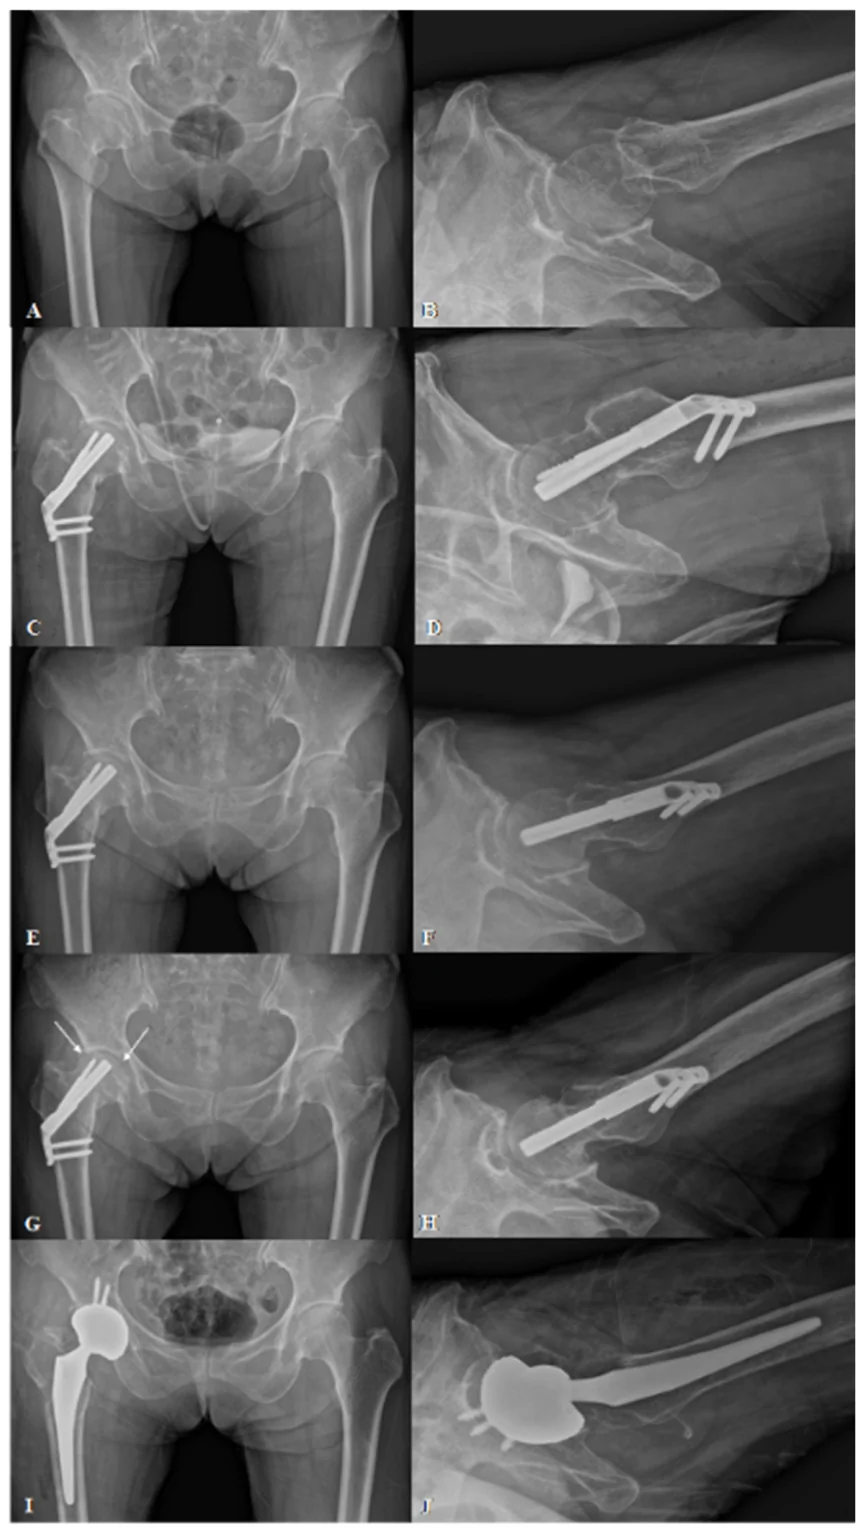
A 63-year-old female sustained a right femoral neck fracture following a ground-level fall. Initial anteroposterior (A) and lateral (B) radiographs show a displaced, complete subcapital fracture. Closed reduction and internal fixation with the Femoral Neck System (FNS) was performed on the day of injury. Immediate postoperative anteroposterior (C) and lateral (D) radiographs demonstrate satisfactory reduction and proper implant placement. At six months postoperatively, anteroposterior (E) and lateral (F) radiographs show radiographic evidence of fracture union. At 10 months postoperatively, the patient developed right hip pain. Anteroposterior radiograph (G) reveals femoral head collapse and flattening (white arrows), indicating osteonecrosis of the femoral head (ONFH), while the lateral radiograph (H) shows relatively less collapse. Due to progressive symptoms limiting daily activities, the patient underwent revision surgery. Anteroposterior (I) and lateral (J) radiographs after revision with total hip arthroplasty.
